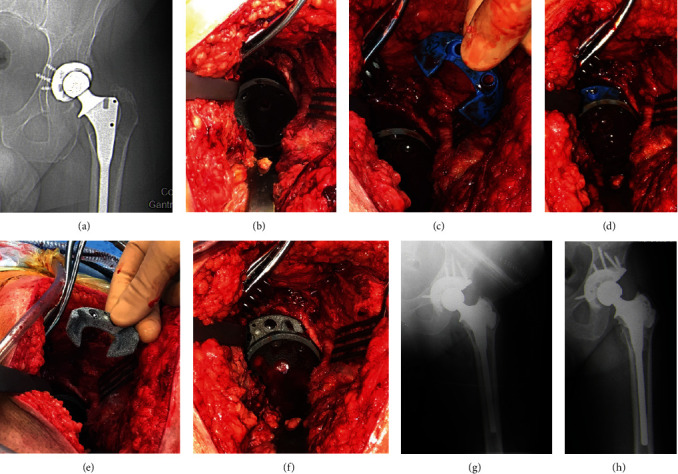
(a) Preoperative X-ray. (b) Intraoperative image after insertion of the cup. (c) and (d) Planification of augment size with trial implants. (e) and (f) Insertion of augment implant (“staple” type). (g) Postoperative X-ray. (h) Two-year follow-up X-ray.
